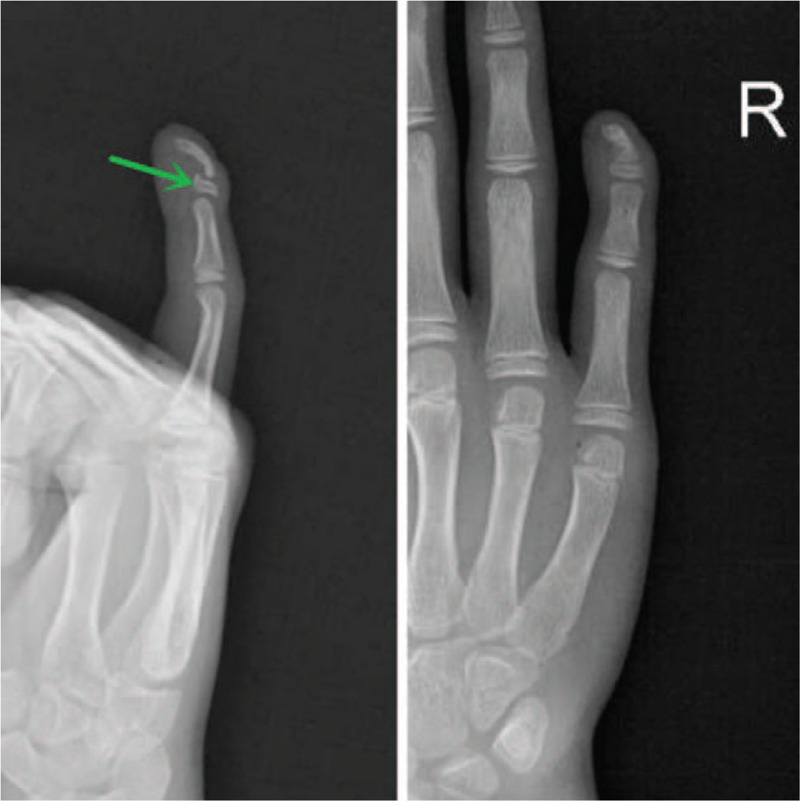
Radiograph shows fifth distal phalanges of the right hand with volar-radial angular deformity, Wider and thicker growth plate of distal phalanx in the form of L-physis (lateral projection).
